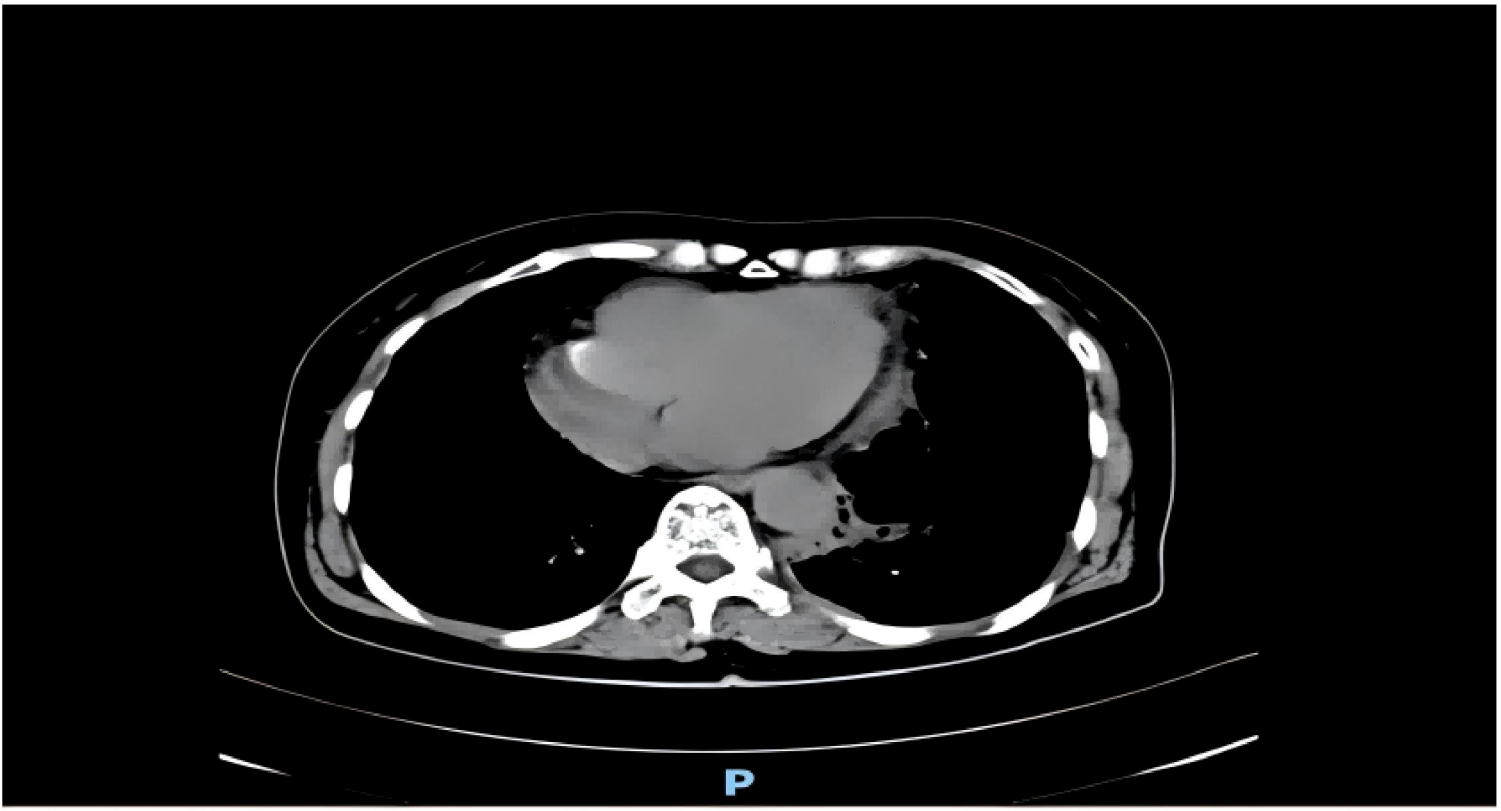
Chest computed tomography of the patient before the operation. There was a massive mass in the anterior mediastinum.(2023 3.24).
